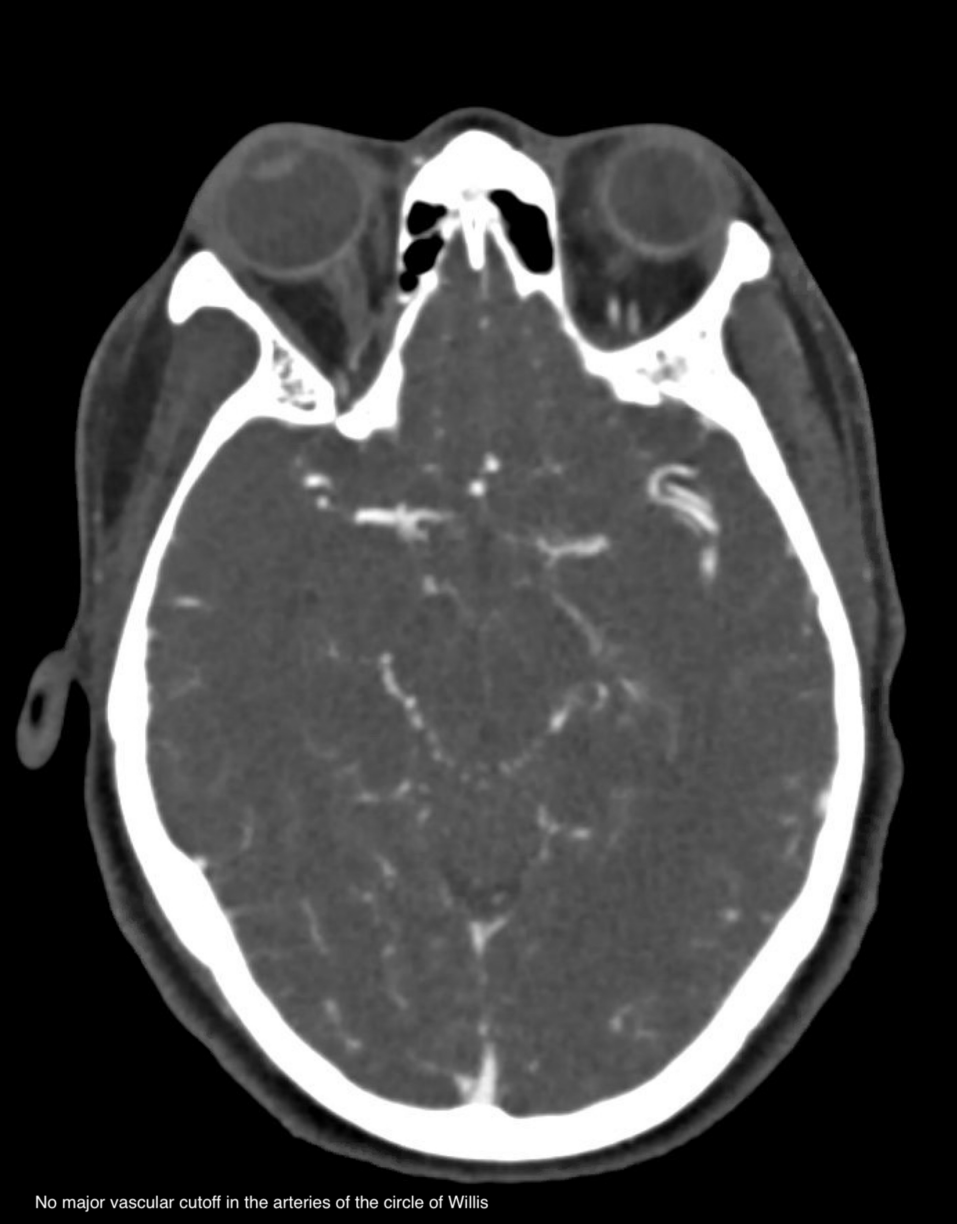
Computed tomography angiography (CTA) head and neck
